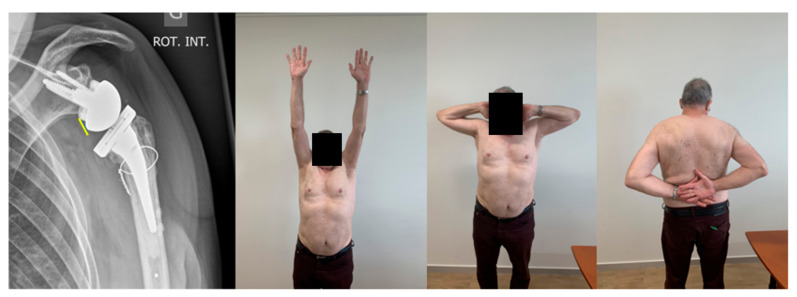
Lateralized RSA after ORIF (Open Reduction Internal Fixation)/osteonecrosis in a young patient after a fracture dislocation and plating. The revision required humeral canal opening with a burr. Outstanding ROM. Radiograph: Combined humeral and glenoid lateralization (Bipolar lateralization; 145° stem, forced varus implantation as a result of sclerosis/insufficient bone stock). Yellow line: Augmented distance from the insert to the lateral scapular margin.
